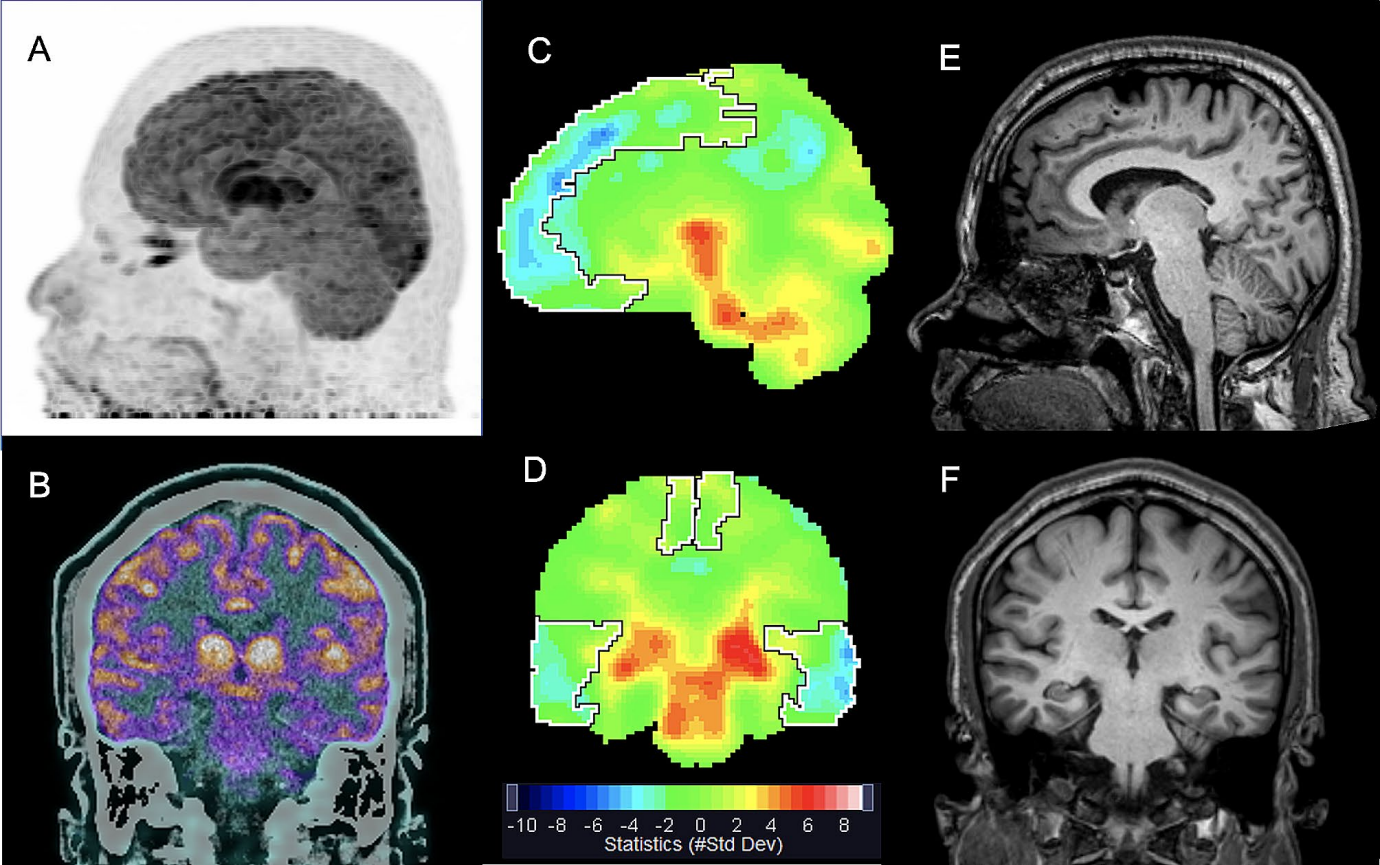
[^18^F]FDG PET/CT of a 43-year-old man on the 12th day of acute COVID-19, with respiratory failure, anosmia and ageusia and no other neurological symptoms. Maximum intensity projection (MIP) (A) and coronal PET/CT image fusion of the brain (B) show marked bilateral [^18^F]FDG uptake in lenticular nuclei and thalami. Quantitative data are displayed in a rainbow color scale as standard deviation (#StdDev) of the mean uptake of a normal database in C (sagittal slice) and D (coronal slice), including the automatic delimitation of frontal and temporal lobes. Note the reduced metabolism in the frontal and temporal lobes (blue in C and D) and increased metabolism in thalami and brainstem (red in C and D), with an appearance of redistribution of [^18^F]FDG uptake from the neocortex to primitive brain structures. An MRI performed three days after PET/CT was totally normal, as seen in sagittal (E) and coronal (F) T1-weighted slices. The patient was followed-up for 12 months and no neurological symptoms or sequelae were detected. Quantification results (in #StdDev units): -2.9 / -3.9, for right / left frontal lobes; -2.0 / -2.4, for right / left temporal lobes; 5.6 / 6.0, for right / left thalami; 4.6, for brainstem
